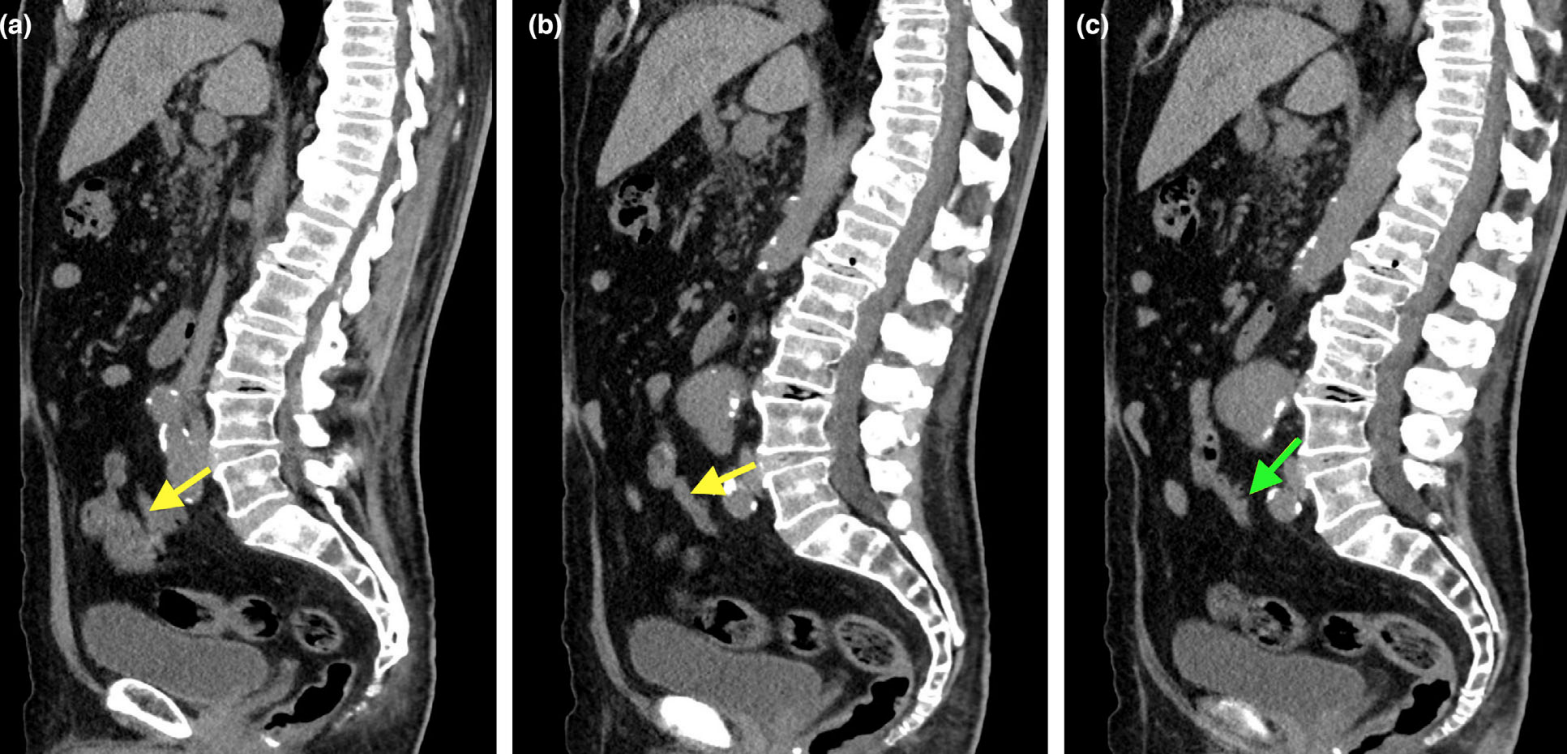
Serial non‐contrast sagittal CT appearance of the patient’s appendix (yellow arrow in A, B) 2 years prior, with small diverticula (green arrow in C), with no features of active inflammation.
